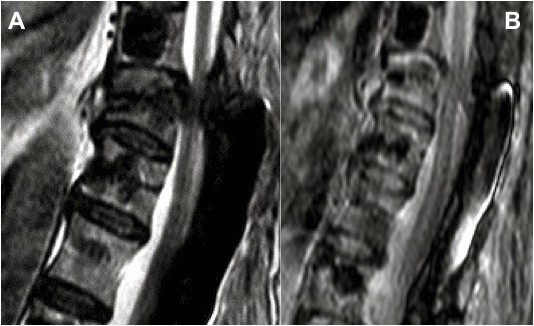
Two sagittal MRI views showing rupture of posterior elements at T9-T10 with high signal intensity and abnormal intracanal tissue compressing the spinal cord.
